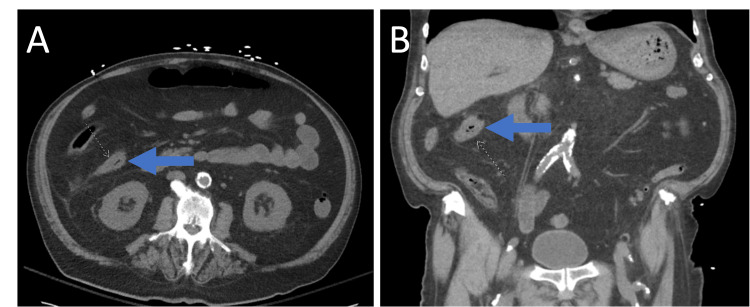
Computed Tomography (CT) of the Abdomen Showing Ascending Colonic Wall Thickening. Arrows pointing to the thickened wall of the ascending colon. Axial (left) and coronal (right) views are shown.
